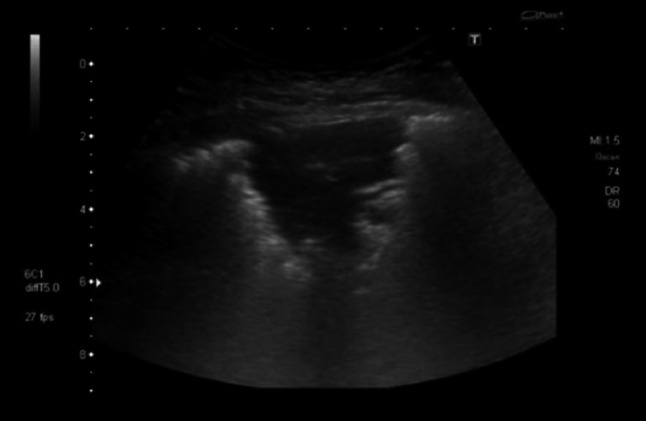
Transthoracic ultrasonography showing a subpleural hypoechoic consolidation. Due to the shredded, fractal boundary between the consolidation and the underlying aerated lung this is called “shred sign”
